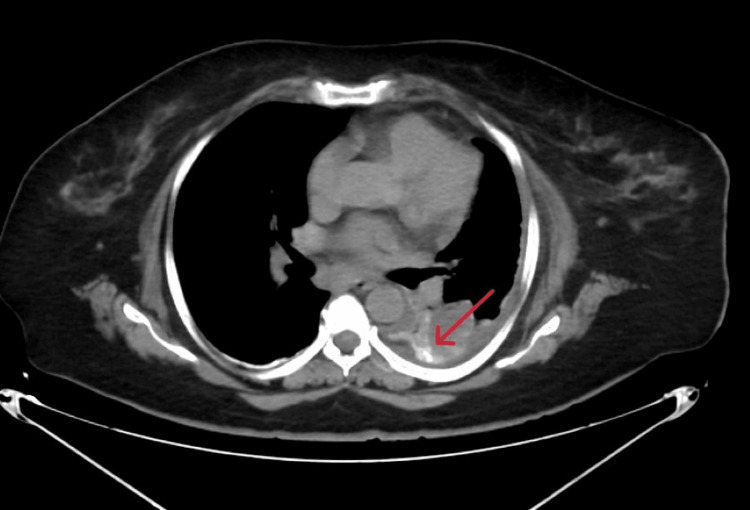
CT of the chest demonstrating contrast accumulation within the left pleural cavity (arrow), representing extravasation following lymphangiography and confirming a thoracic duct leak.
